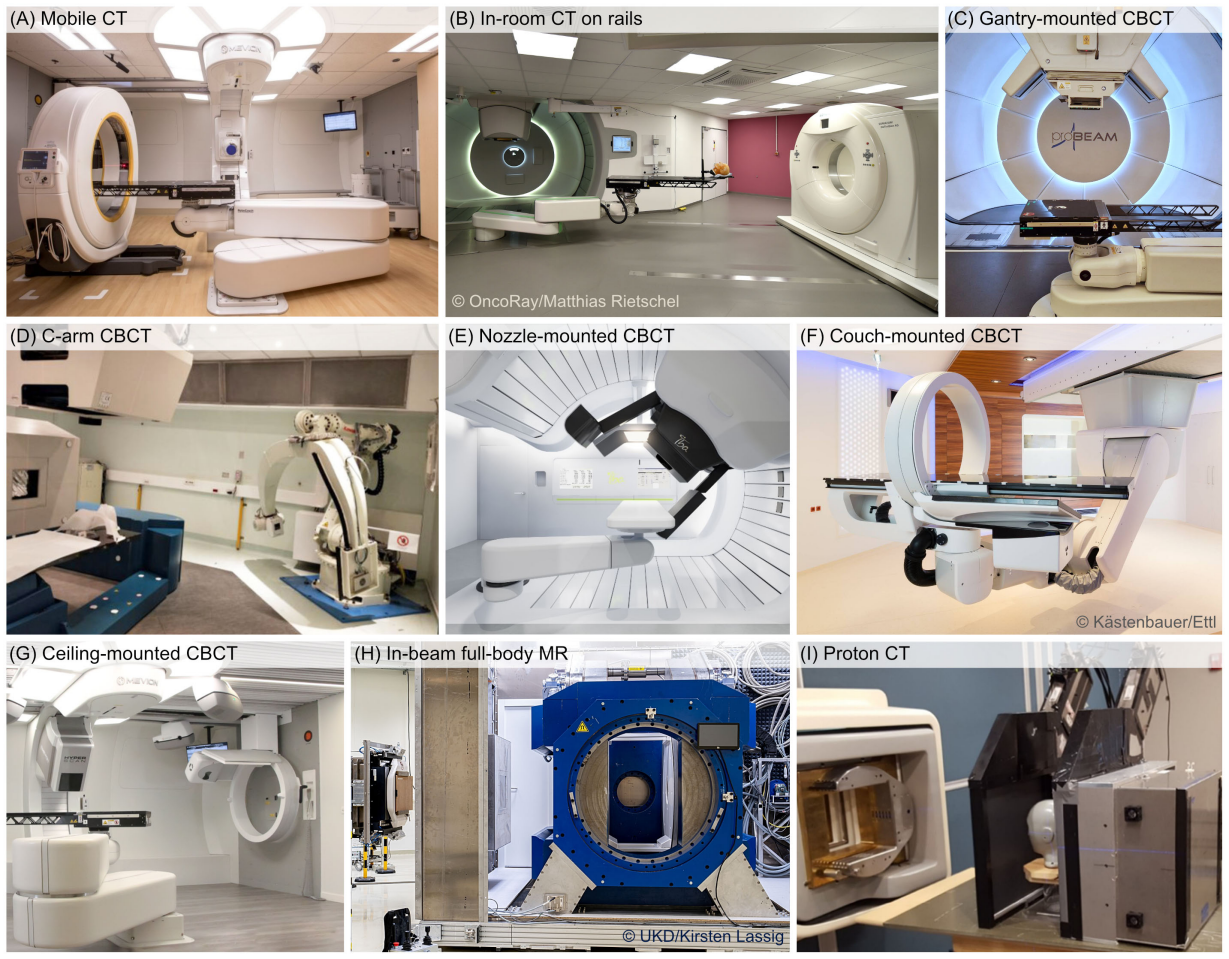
Overview of discussed volumetric imaging solution for treatment planning: (A) mobile CT scanner (reproduced from https://www.itnonline.com/content/orlando-health-and-mevion-offer-advanced-diagnostic-image-guidance-proton-therapy with permission), (B) Siemens in-room CT on rails at OncoRay (reproduced from https://www.hzdr.de/db/Cms?pOid=43592&pNid=99 with permission), (C) Varian gantry-mounted CBCT at Paul Scherrer Institute, (D) CNAO C-arm mounted CBCT (reproduced from (36) under CC-BY 4.0 license), (E) IBA nozzle-mounted CBCT (reproduced from https://www.itnonline.com/content/iba-and-philips-step-commercial-collaboration-brazilian-proton-therapy-market with permission), (F) Medphoton (Brainlab) couch-mounted CBCT at MedAustron, (G) Medphoton (Brainlab) ceiling-mounted CBCT at Maastro Proton Therapy (courtesy of Mevion Medical Systems and Maastro Proton Therapy), (H) full-body, rotatable MR scanner integrated at horizontal beamline at OncoRay, (reproduced from https://www.hzdr.de/db/Cms?pOid=71010&pNid=0 with permission) (I) experimental proton CT prototype (reproduced from (35) under a CC-BY 4.0 license).
